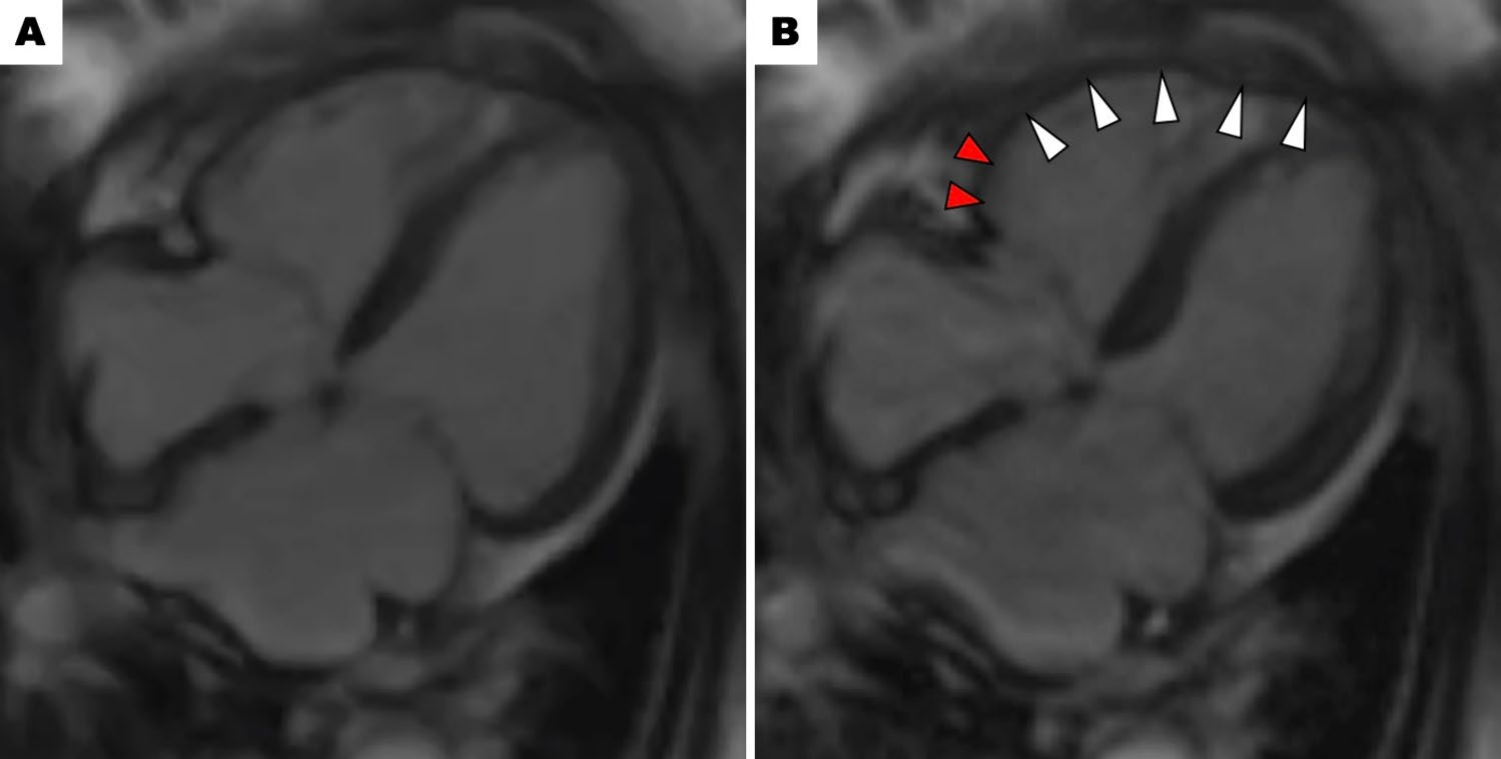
Right ventricular involvement. Cardiac magnetic resonance imaging demonstrates ballooning involving mid to apical segments (white arrows) and hyperkinetic basal segments (red arrows) of the right ventricle. Panels A (diastole) and B (systole)TTS is a disease that primarily presents with wall motion abnormalities in the LV, but in some cases, wall motion abnormalities are also apparent in the right ventricle (RV) [16]. Previous reports demonstrated that the prevalence of RV involvement is approximately 10–50% [17–21]. This variation may be due to the small number of cases, differences in the definition of RV wall motion abnormalities, and differences in imaging evaluation methods. Kagiyama et al. reported from an echocardiographic study that 18.6% of patients with TTS showed RV involvement, and patients with RV involvement more frequently experienced cardiogenic shock [18]. El-Battrawy et al. demonstrated that the incidence of RV involvement detected by echocardiography or cardiac magnetic resonance was 11%, and preceding physical trigger was an independent predictor of RV involvement. In addition, patients with RV involvement had a higher incidence of cardiogenic shock during hospitalization and a worse long-term outcome [19].If RV involvement is the primary cause or an exacerbating factor of cardiogenic shock in TTS, fluid administration is the first-line treatment. Agents that reduce preload, like diuretics and nitroglycerin, are not recommended as they can further compromise hemodynamics. If fluid administration is ineffective for improving hemodynamic status, additional use of inotropic agents might be considered. Non-catecholaminergic inotropic agents like levosimendan and milrinone are preferred in the treatment of TTS, but such agents should be used with caution in patients with RV involvement because of their potential vasodilatory effects that could worsen RV preload. In cases of persistent hypotension despite improved contractility, vasopressors like norepinephrine or vasopressin may be necessary. LVOTO (Fig. 4)
